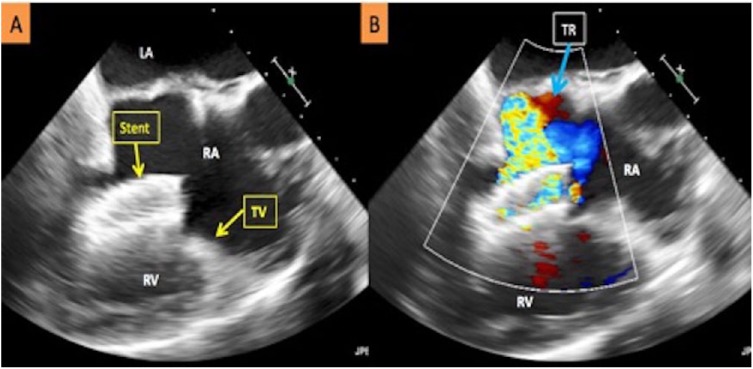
(A) Transesophageal echocardiogram depicting the migrated stent across the tricuspid valve resulting in severe tricuspid regurgitation. (B) Doppler-enhanced transesophageal echocardiogram image depicting severe tricuspid regurgitation as a result of the migrated stent. LA, left atrium; RA, right atrium; RV, right ventricle; TV, tricuspid valve; TR, tricuspid regurgitation.
